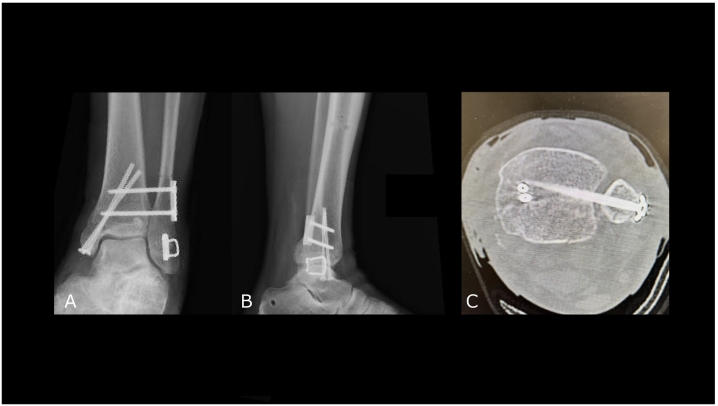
Postoperative findings. A) Anteroposterior X-ray of the ankle. B) Lateral X-ray of the ankle. C) Axial CT view of the distal tibiofibular joint. The proper reduction of the tibiofibular syndesmosis was obtained.
